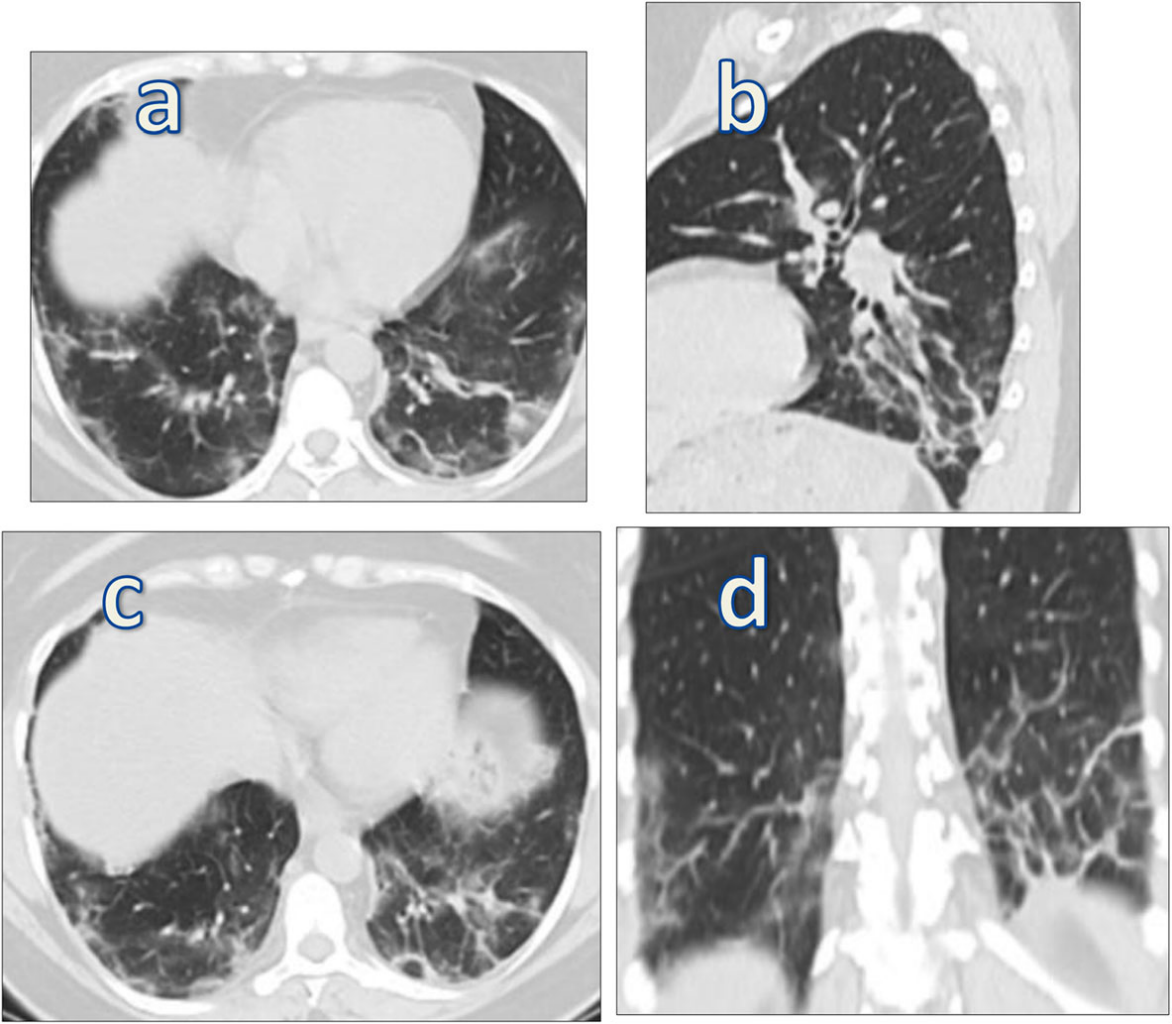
A 51-year-old female patient presented with dyspnea and dry cough, proved to be COVID-19 (positive by PCR). CT-SS on admision was 12/25. Follow-up MDCT chest (axial, sagittal, and coronal reconstructive images) after 4 weeks (a, b) revealed bilateral lower lobar fibrotic changes with thick parenchymal bands, subpleural lines, and traction bronchiactasis and bronchiolectasis, which became more extensive in follow-up films at 12 weeks (c, d)
